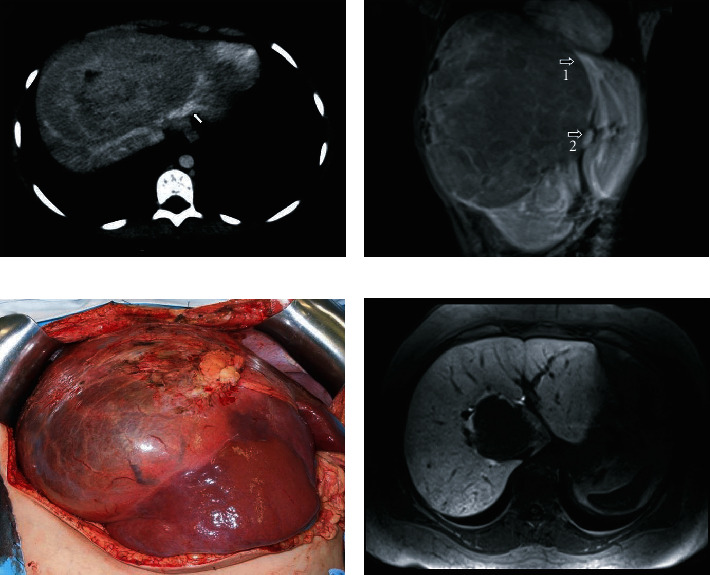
(a) MRI images showing a large heterogeneous mass compromising the RL and segment IV and in close contact with the FLR vessels (Arrow). (b) MRI image showing a large heterogeneous lesion occupying the whole RL and preserving segments II, III, and inferior IV. The left hepatic vein (arrow 1) and the left branch of the portal vein (arrow 2) are in close contact with the lesion. (c) Intraoperative aspect: tumor mass occupying almost the entire liver, preserving only segments II, III, and inferior IV. (d) MRI with hepatospecific contrast agent showing a lesion without contrast enhancement on the hepatobiliary phase in the caudate lobe with involvement of the middle and right hepatic veins and in close contact with the left hepatic vein.
